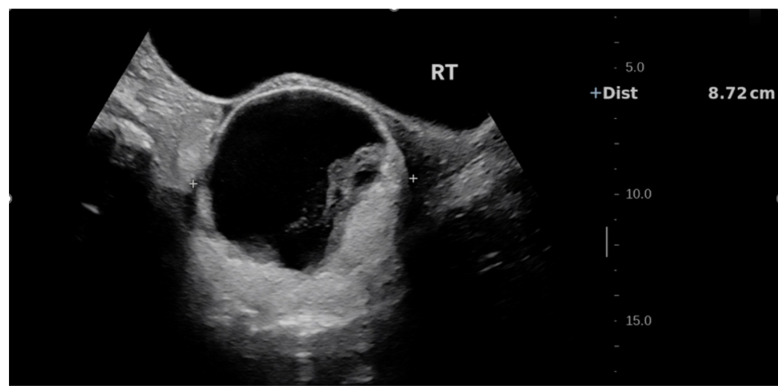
Transabdominal pelvic ultrasound revealed a right adnexal cystic–solid mass measuring 8.4 × 8.7 × 8.5 cm, demonstrating a mixed cystic–solid pelvic mass in the right adnexal region. The center of the lesion is predominantly cystic with anechoic areas. The lesion shows irregular internal walls with patchy hyperechoic areas. The right ovary is not clearly visualized.
